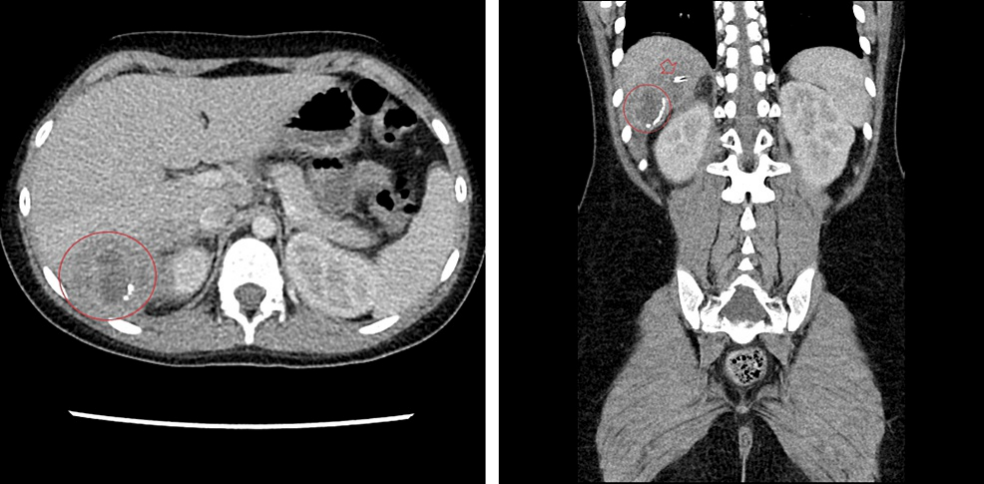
Computed tomography image showing a hepatic abscess taken on the third day of admission. Along the inferomedial border of the lesion in the coronal view, increasing levels of calcifications can be seen forming a radiolucent border. Superomedial to the lesion, an area of radiolucency is seen (arrow); while previously ambiguous on imaging, it appears to be clip-like in shape, but clinical history makes this unlikely.
